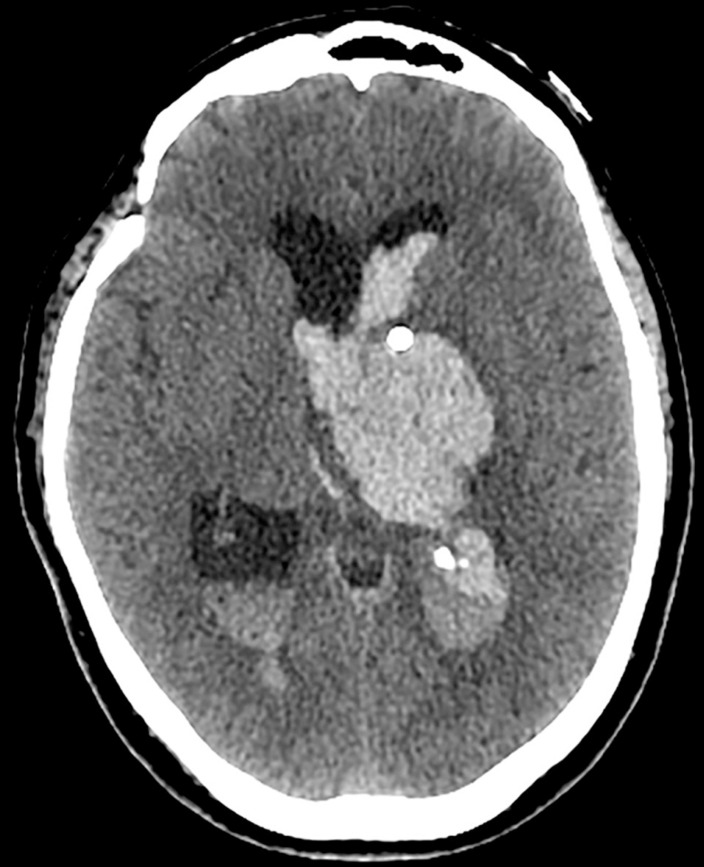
Head CT reveals an intracerebral hemorrhage of approximately 50
